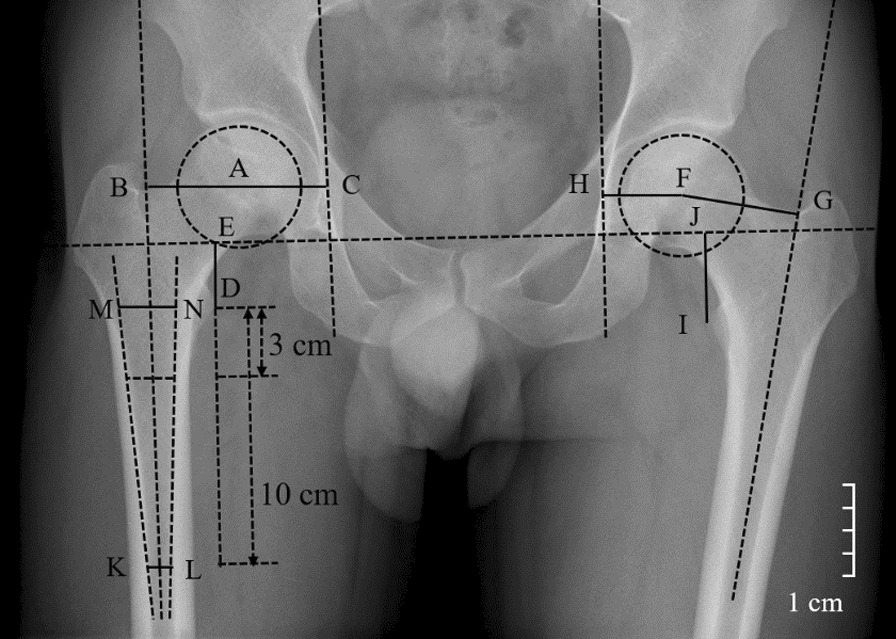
Radiographic measurement. The calcar width was measured as the middle level of the lesser trochanter (line MN). The canal width was measured as 10 cm below the lesser trochanter (line KL). The calcar-to-canal ratio was calculated as line KL divided by line MN, which was used for the Dorr classification. The femoral offset (FO) was measured as the vertical distance from the center of COR and the ipsilateral anatomical femoral axis (line AB and line FG). The acetabular offset (AO) was measured as the vertical distance from the COR and the vertical line passing through the ipsilateral teardrop (line AC and line FH). The hip offset (HO) was calculated as the sum of FO and AO (line AB + line AC and line FG + line FH). Leg length discrepancy (LLD) was calculated as the difference between the bilateral vertical line from the most prominent part of the trochanter to the transteardrop line (difference of line ED and line JI)
